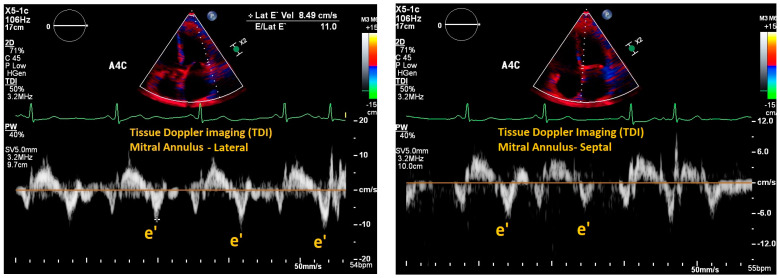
Lateral and septal mitral annular e’ velocities using tissue Doppler imaging (TDI). The e’ velocity corresponds to the velocity of tissue relaxation in early diastole. The average of lateral and septal mitral e’ velocities is used to calculate the E/e’ ratio which can be used to estimate LAP.
